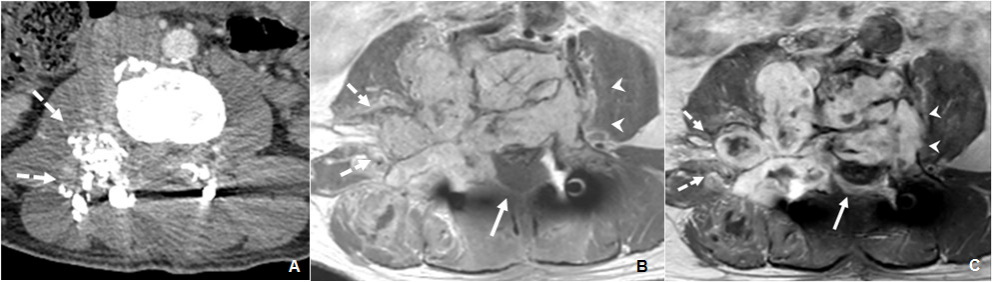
A*. Post-procedure arterial-phase contrast-enhanced CT showing the embolized extradural component of the haemangioblastoma, with hyperdense embolization material (dashed arrows). *B*. Axial *T*_1_ weighted contrast-enhanced MRI 1 month after the percutaneous embolization and excision, showing satisfactory surgical decompression of the intradural component with reduction in spinal canal stenosis (solid arrow). Laminectomy at this level was also present with metallic surgical rods and associated susceptibility artefacts. *C*. Axial *T*_1_ weighted contrast-enhanced MRI 6 months after percutaneous embolization and excision, demonstrating satisfactory surgical decompression of the intradural component with reduction of spinal canal stenosis. Mild interval growth is present in the left paraspinal region (arrowheads), while the embolized right paraspinal component (dashed arrows) is static in size.
